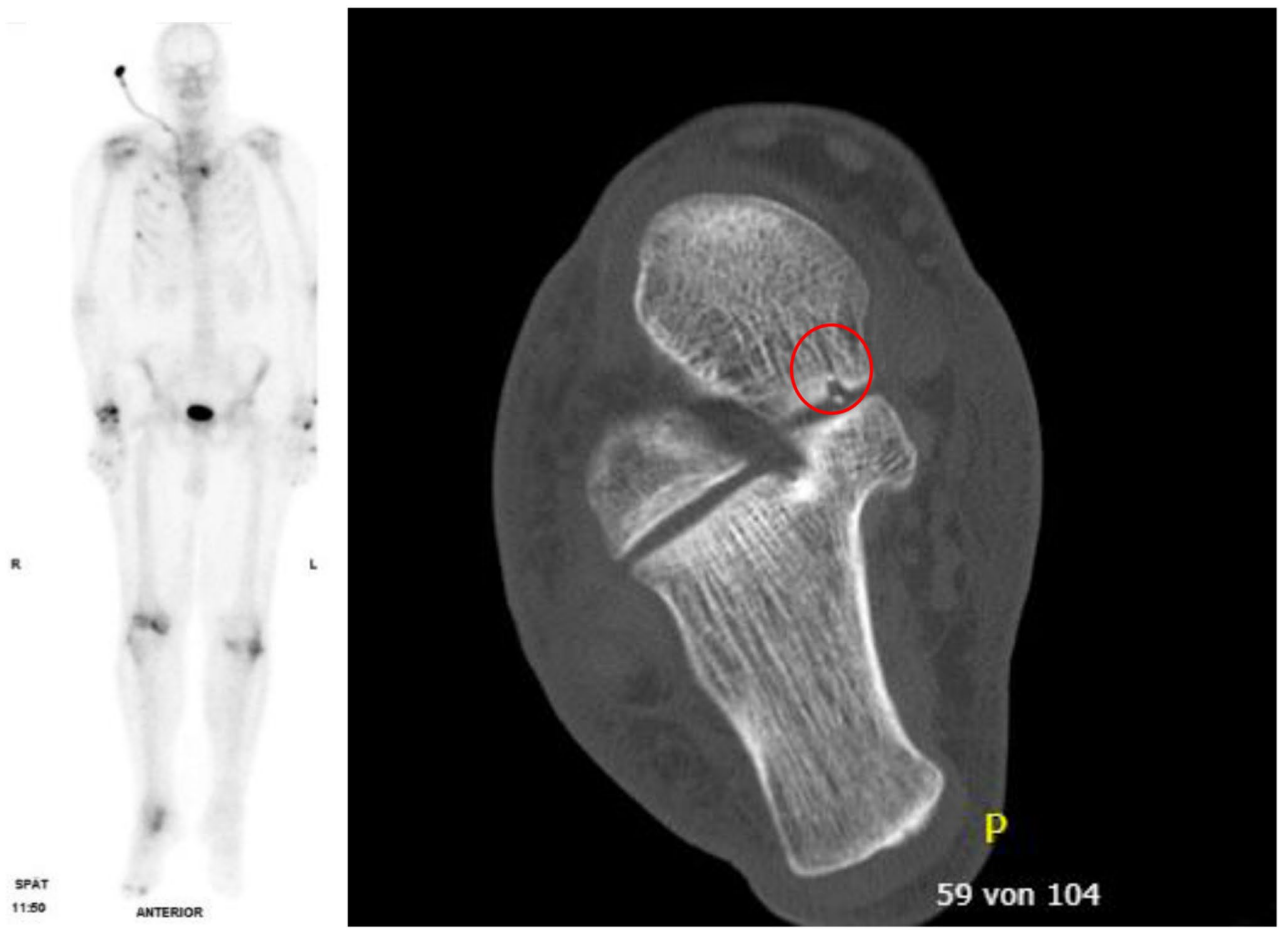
Late phase of the scintigraphy. Various rib fractures on the right and, among other features, an accumulation in the right talus can be seen well. A talus fracture (circle) that was barely visible on the CT was confirmed here
